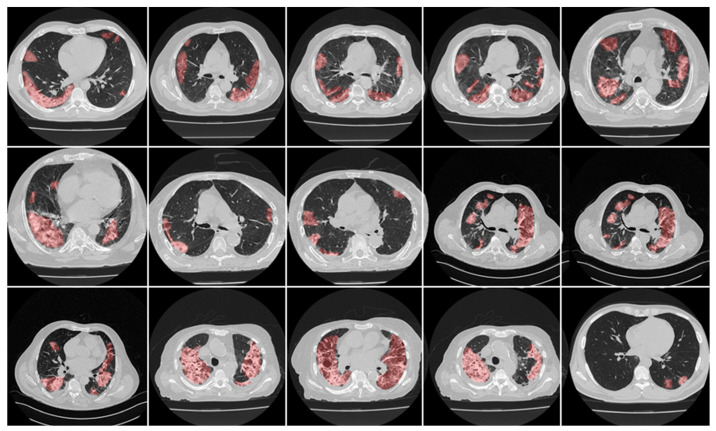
Manual delineation overlays (red) from tracer 1 on raw CT images.
